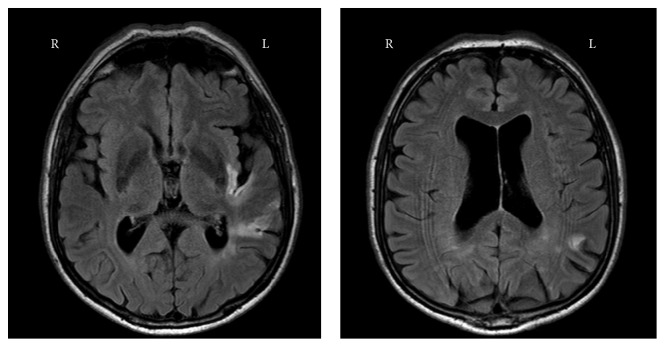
Transversal fluid attenuated inversion recovery (FLAIR) images of the patient, revealing a lesion to the posterior part of the insula and part of the left temporal cortex and left parietal cortex.
